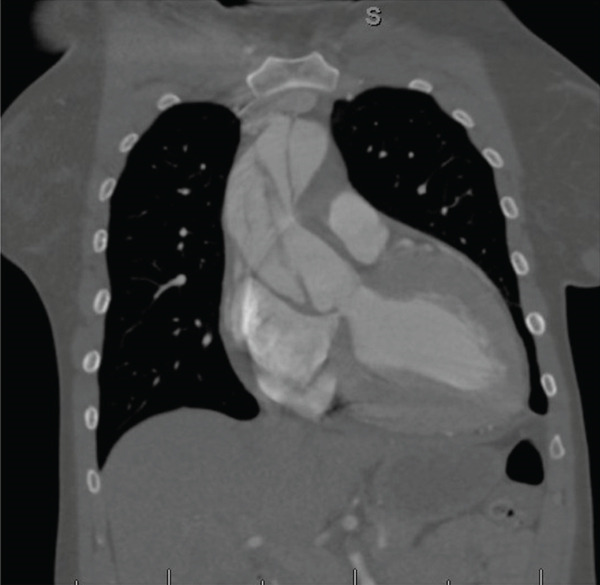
Coronal CT chest demonstrating Stanford Type A aortic dissection.
